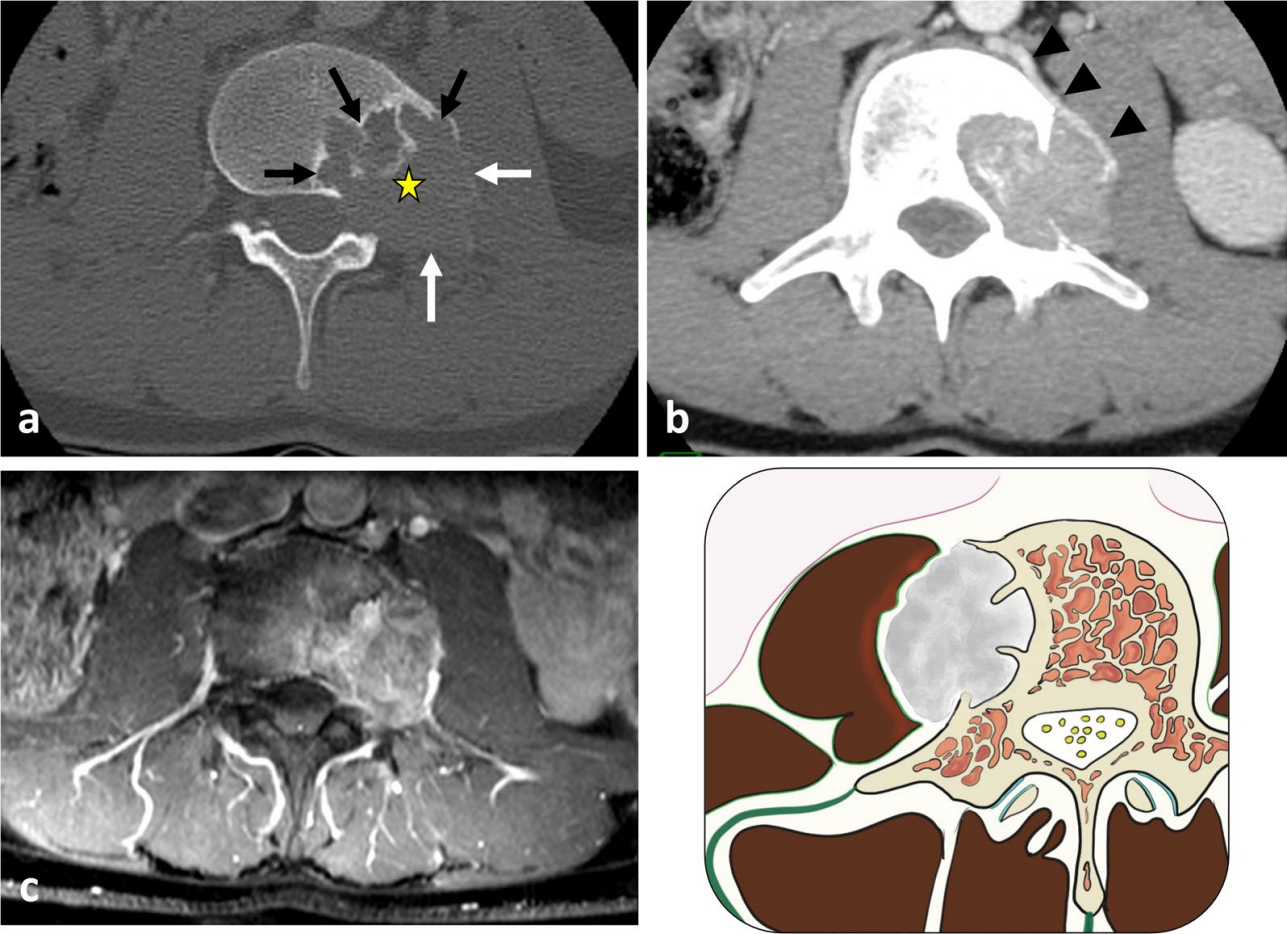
Giant cell tumour* with left corporeal-pedicle development in a 42-year-old female with low back pain of 4 months of duration (a, b, c). Axial CT scan reconstruction (a) shows a lytic lesion with a sclerotic rim (black arrow) at the border of the tumour opposite to the eccentric side. As shown on axial CT with contrast agent (b), the tumour typically splays rather than engulfs the psoas muscles (white arrows) and the lumbar artery (arrowheads). Axial gadolinium-enhanced T1WI (c) demonstrates homogeneous and moderate enhancement. Yellow star shows the epicentre of the mass
